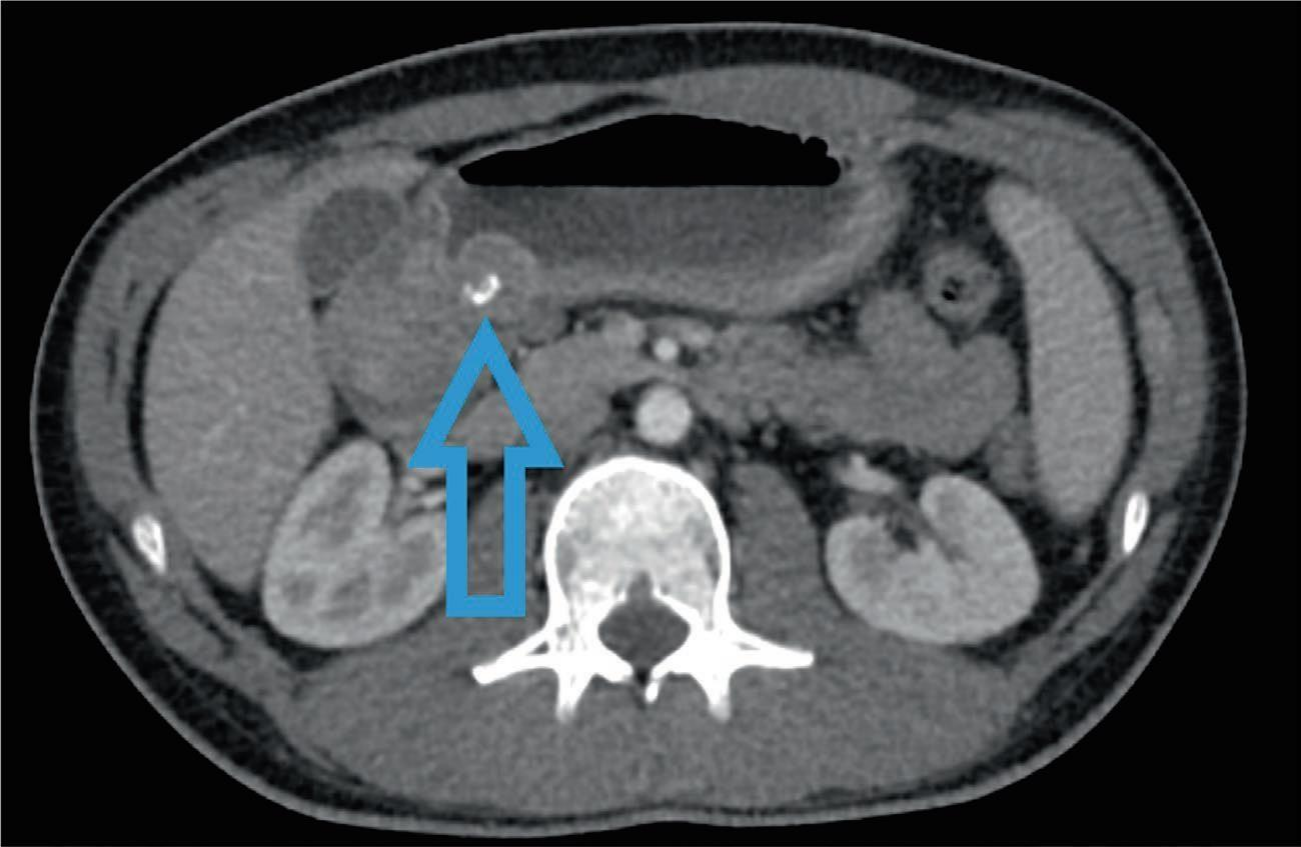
Computed tomography imaging revealed obstruction of the pyloric outlet by a 2.4 cm mass with associated calcifications (blue arrow points to calcifications).
